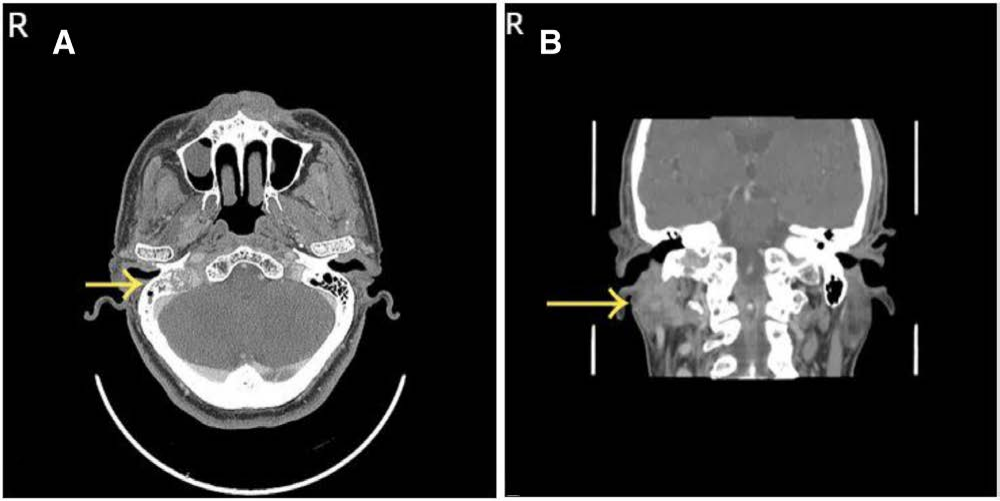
(A, B) Enhanced CT of temporal bone showed the petrous cone of the right temporal bone showed irregular enhancement density and osteolytic destruction. CT = xxx.
